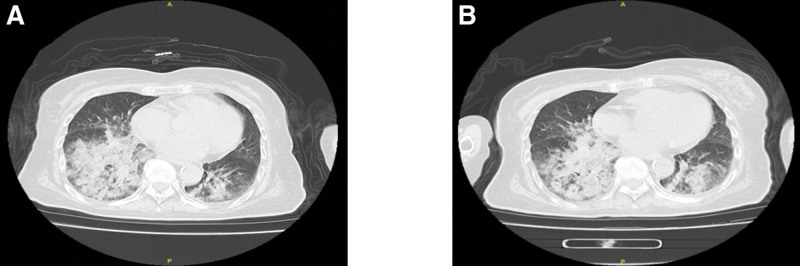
Chest computed tomography changes in the patient’s lungs at the end of the second treatment cycle. (A) May 10, 2024. (B) May 21, 2024.
